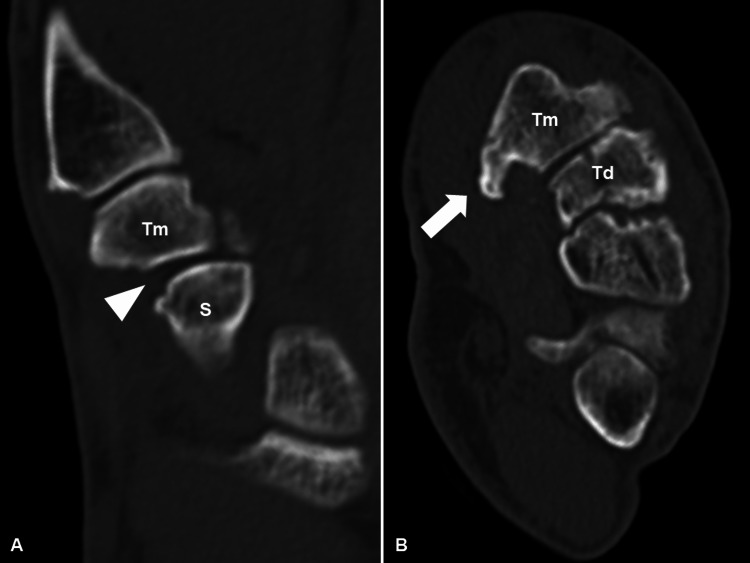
Plain computed tomography at one year postoperatively. (A) Coronal and (B) axial CT images (Arrowhead shows joint space of the scaphotrapeziotrapezoid joint, and arrow shows bony union of the volar fragment of the trapezium). S, scaphoid; Td, trapezoid; Tm, trapezium
